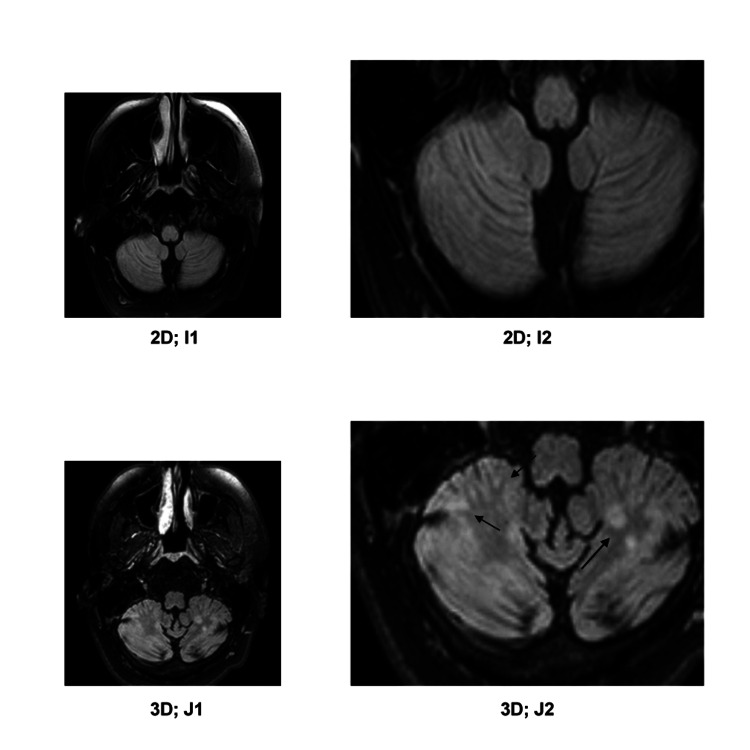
I1) 2D-FLAIR image; I2) detailed enlargement of I1; J1) 3D-FLAIR image, roughly aligning with I1; J2) detailed enlargement of J1. On the 3D-FLAIR images, six lesions in the bilateral-cerebellar hemispheres (marked with arrows in J2) are visible, which are undetected on the 2D-FLAIR images.
